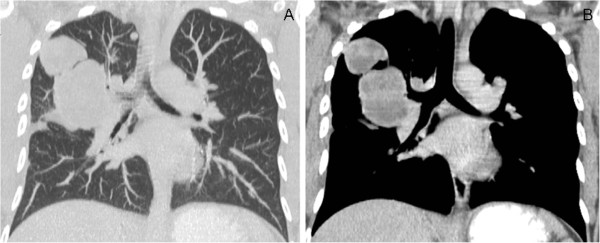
Pulmonary artery angiosarcoma. A and B. CT-chest shows two circumscribed pulmonary masses.
